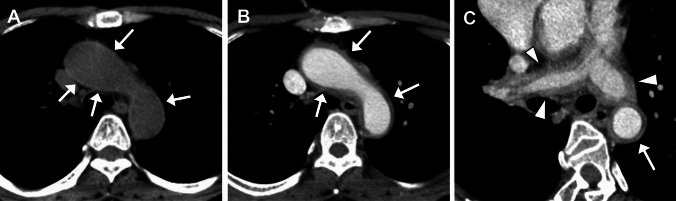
Double-ring sign in Takayasu arteritis. A 49-year-old woman with back pain. (A) Pre-contrast CT shows hyperattenuation along the aortic wall (arrows). (B) Contrast-enhanced CT shows hyperdense outer (arrows) and hypodense inner layers of the aortic wall. (C) An oblique short-axis image of aortic arch clearly shows a concentric ‘double-ring’ appearance (arrow). Note diffuse wall thickening of the pulmonary arteries (arrowheads)
